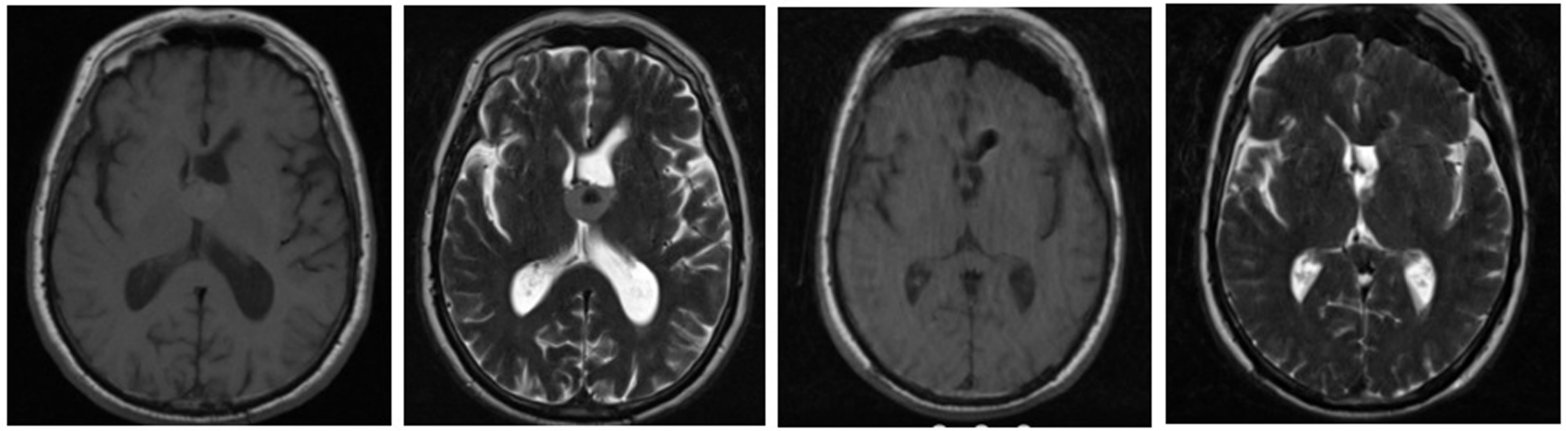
Preoperative and postoperative T1- (T1W) and T2-weighted (T2W) axial images of a patient who underwent total resection.
